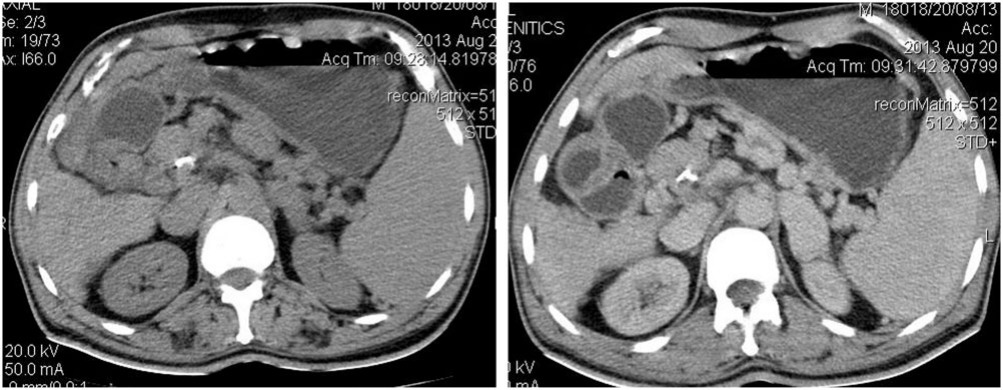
Follow-up CT images showing resolution of the mass. Portal vein calcification is seen persisting in the follow-up image.
